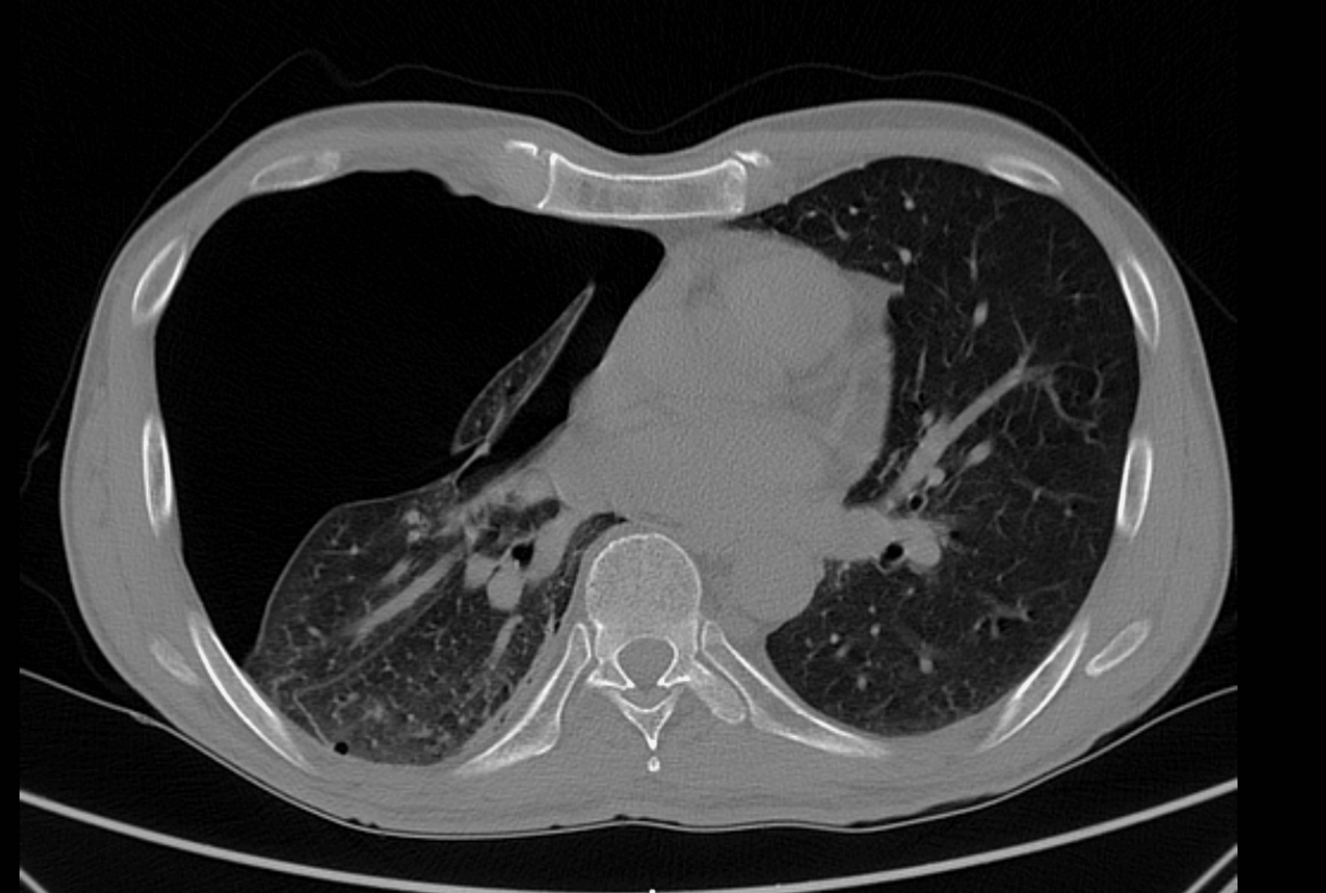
Thoracic computed tomography revealing complete pneumothorax on the right side This thoracic computed tomography showing a complete right-sided pneumothorax with partial lung collapse, without evidence of additional acute parenchymal abnormalities.
